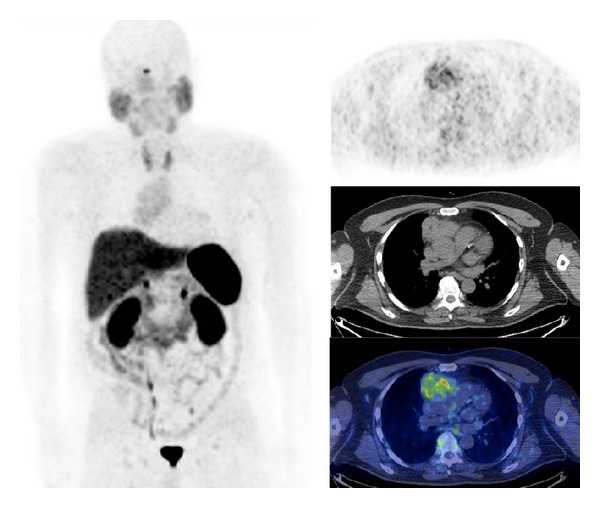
^68^Ga-DOTATATE PET/CT images (MIP, axial) in a patient with thymoma.
